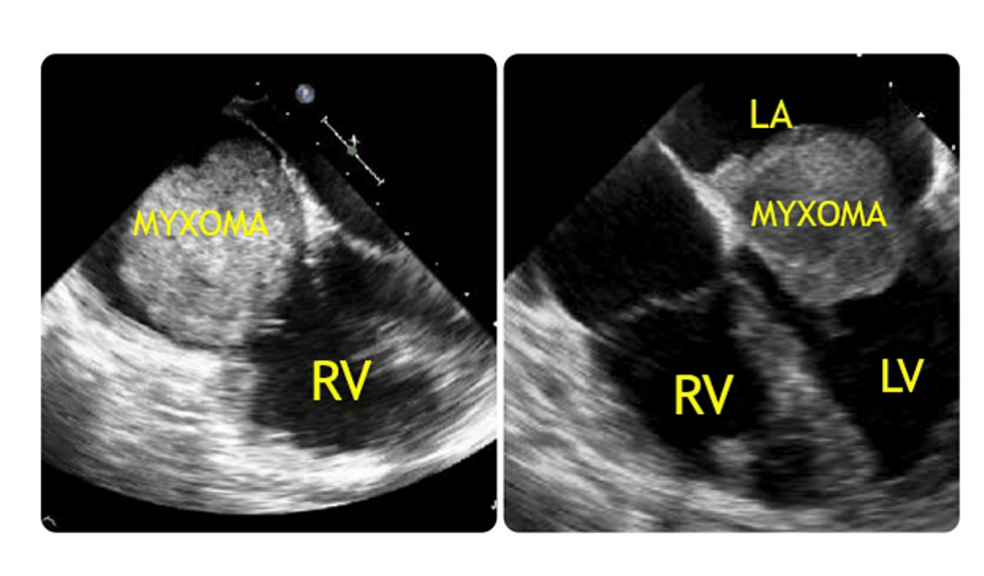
TEE studies showing right and left atrial myxoma, attached to inter-atrial septum. LA: left atrium; LV: left ventricle; RV: right ventricle; TEE: transesophageal echocardiography
